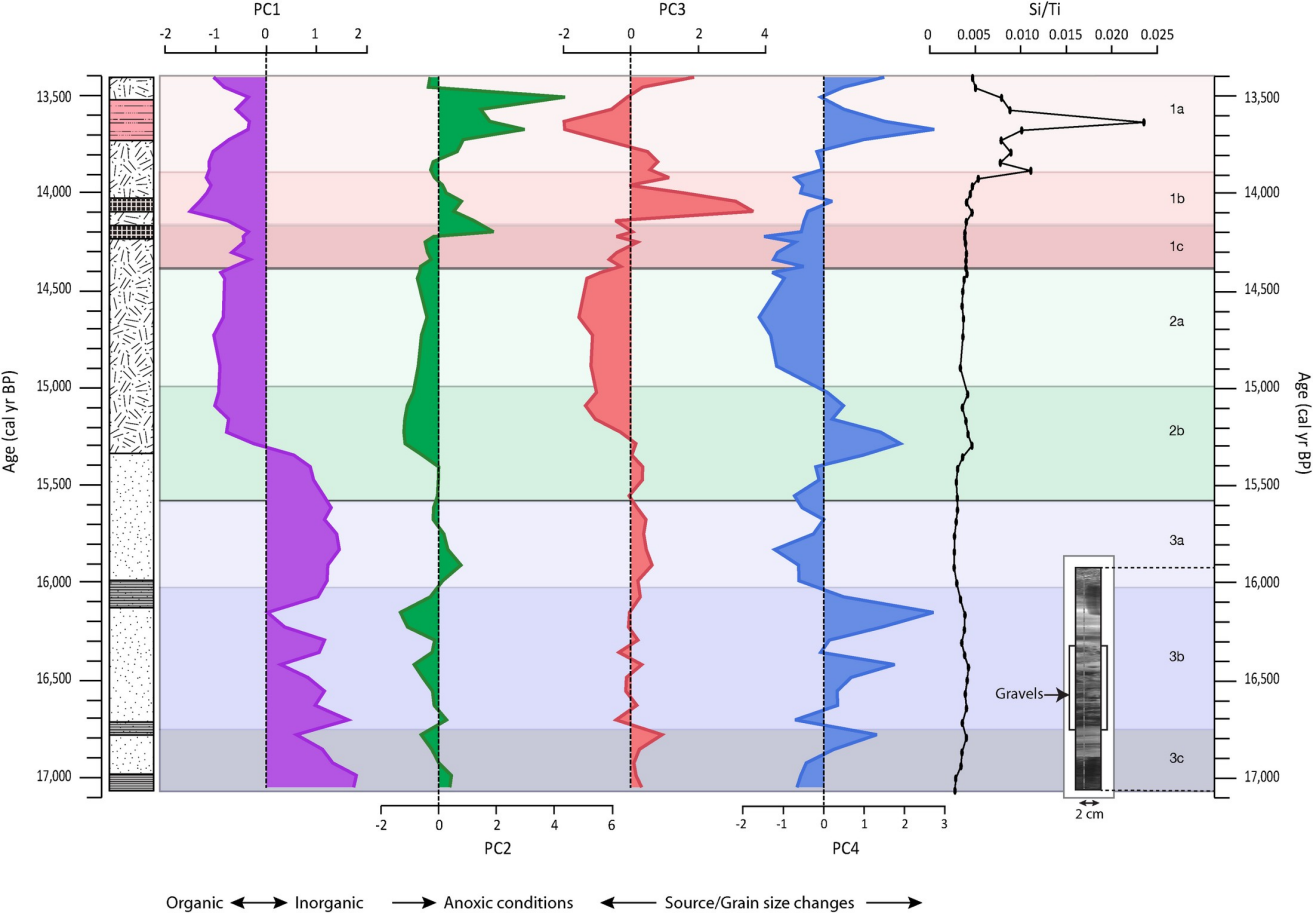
Plots of factor scores by depth of PC1, PC2, PC3 and PC4 as well as Si/Ti ratios by age. The general interpretation of the PC is given. Also included is the available radiograph for the bottommost section which was sampled using a Russian corer, allowing for X-ray scanning.
